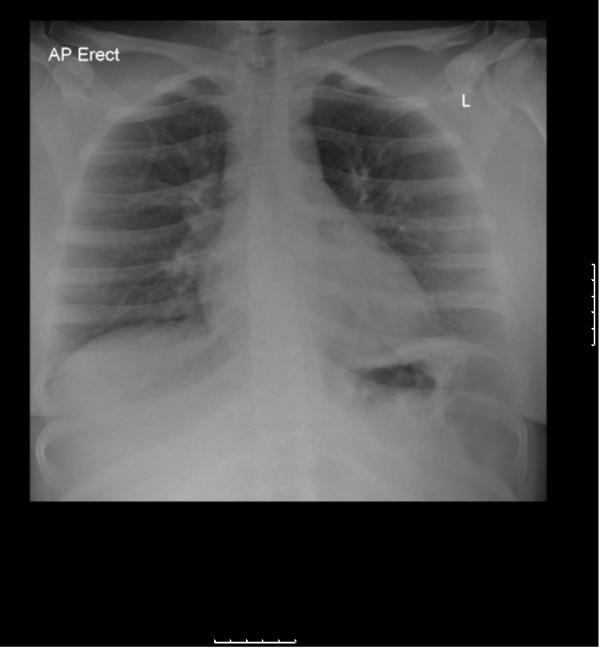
Severe hypoxia requiring noninvasive ventilation is not explained by this relatively normal chest X-ray.
